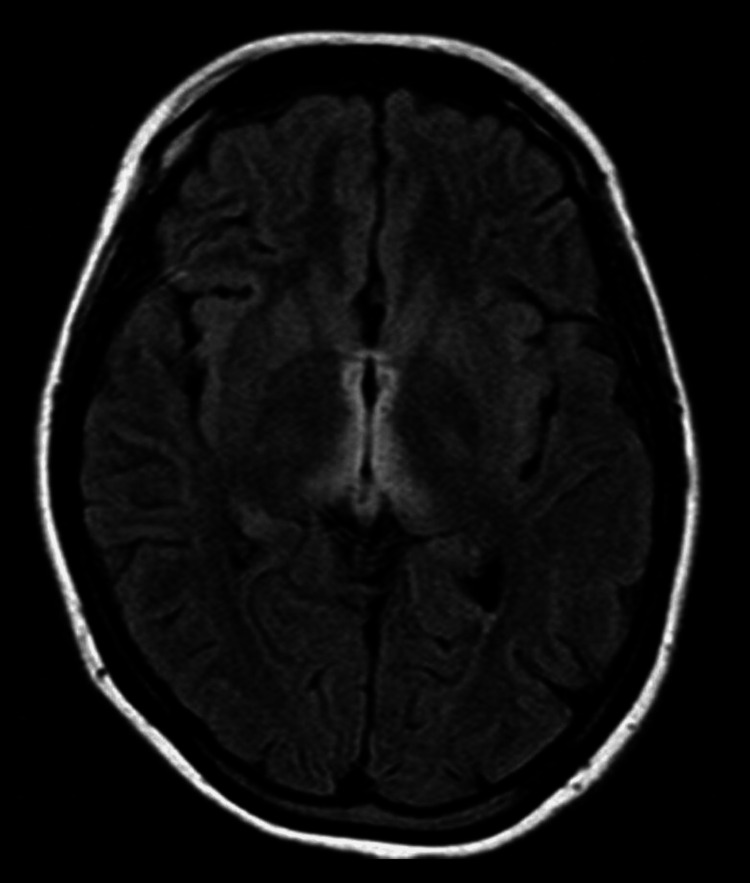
T1-weighted and DWI images showed no alterations DWI: diffusion weighted imaging
